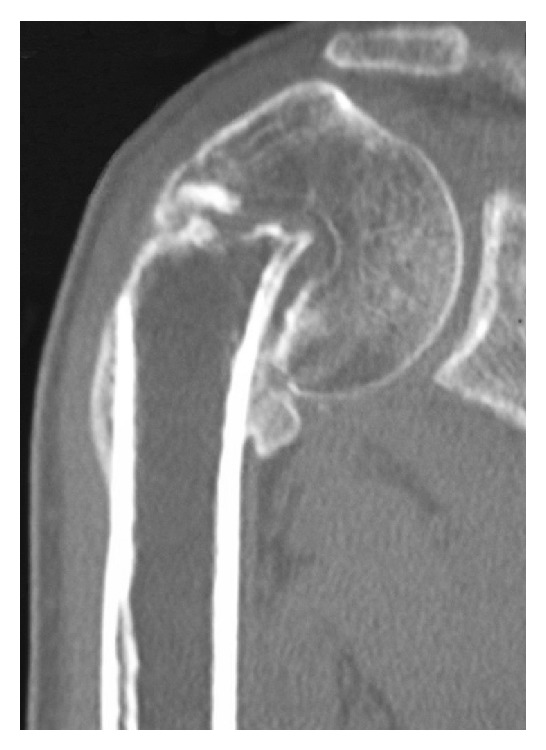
Preoperative CT image (oblique coronal plane) clearly shows that the greater tuberosity locates just beneath the undersurface of acromion.
